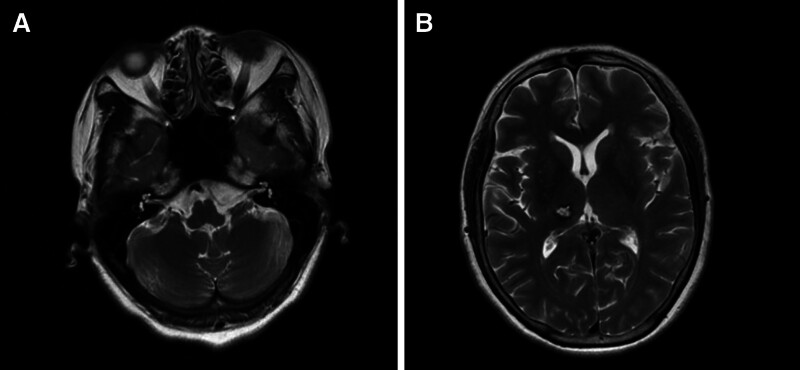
(A, B) Cerebral MRI showed hemorrhages in the right thalamus with hemosiderosis.
